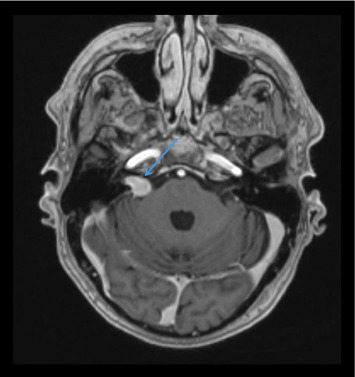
Axial reconstruction postcontrast MRI. White arrowhead shows right vestibular schwannoma with intracanalicular and cisternal components. No significant mass effects on the adjacent brainstem or middle cerebellar peduncle.
